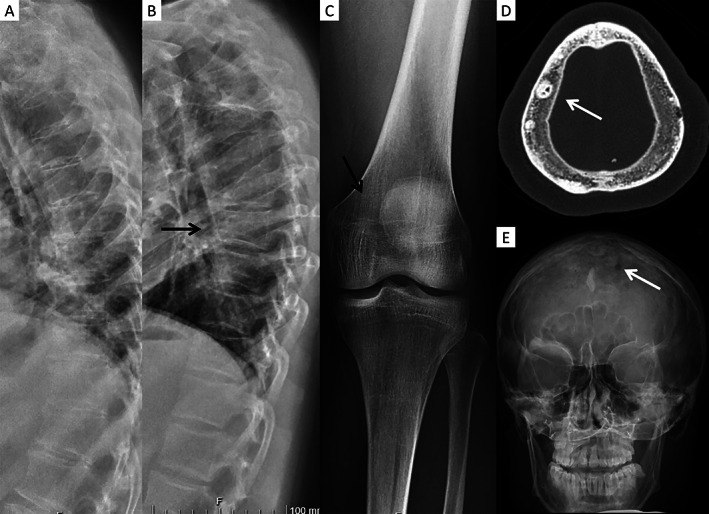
Skeletal radiographs for the two males with *SGMS2* nonsense mutation p.Arg50*. (*A*,*D*) Patient 1, at age 55 years. (*B*,*C*,*E*) Patient 2, at age 27 years. Images show poor and uneven mineralization with visible trabeculation in vertebrae and long bones (black arrows) and multiple sclerotic cranial lesions in skull bones (white arrows).
